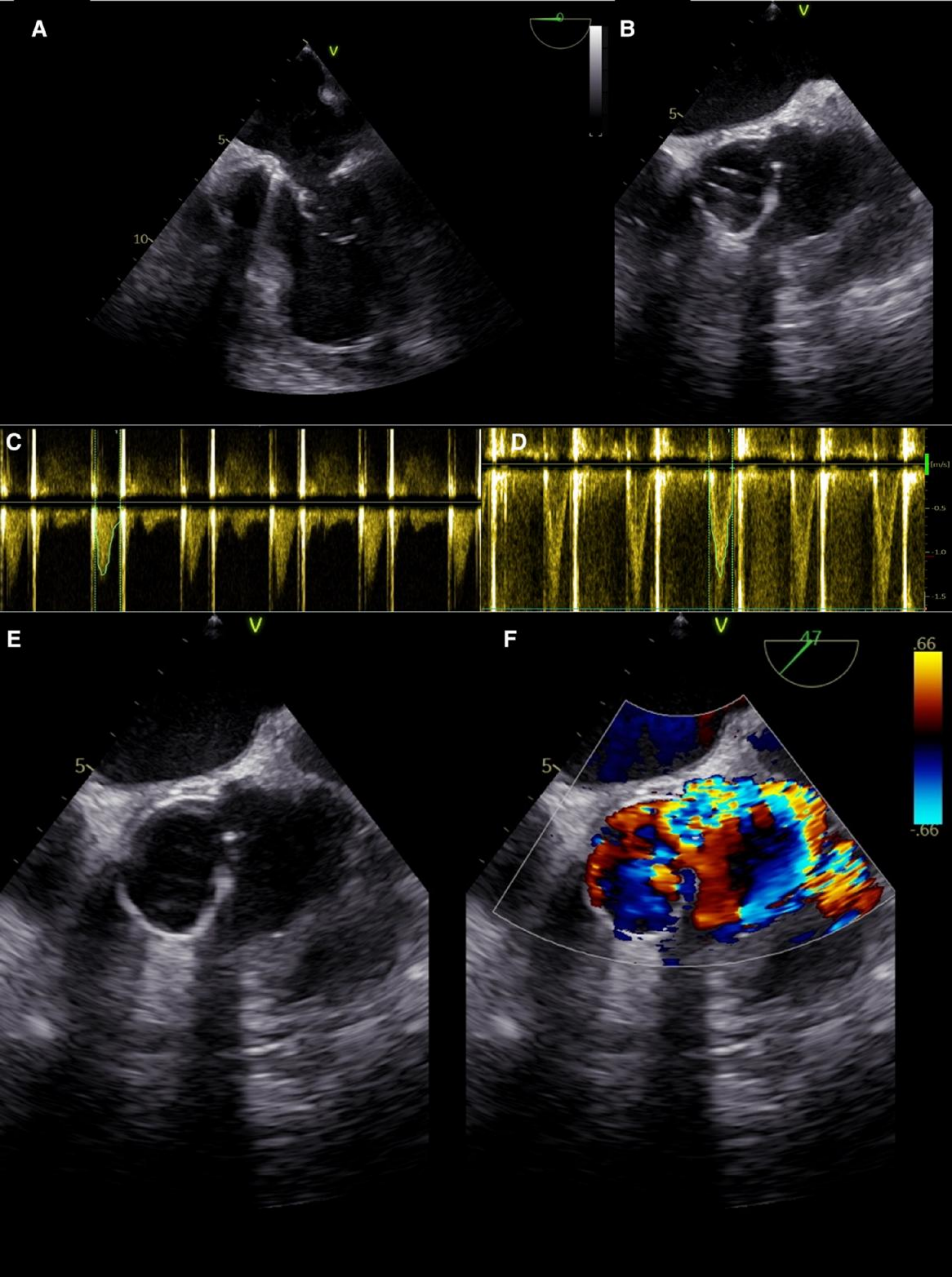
Transthoracic echocardiography and transoesophageal echocardiography images. Transoesophageal bicommisural view (*A*) demonstrating a mild to moderate dilation of the left ventricle. Transoesophageal short-axis view (*B*) showing the mechanical prosthetic aortic valve and the pseudoaneurysm of the aortic root. Continuous and pulsed wave Doppler at transthoracic echocardiography (*C–D*) demonstrating a normally functioning mechanical aortic valve (mean gradient 5.5 mmHg, max. velocity 1.7 m/s, DVI 0.7). Zoomed short-axis transoesophageal view (*E–F*) exhibiting the voluminous pseudoaneurysm with the colour Doppler demonstrating the communication of the pseudoaneurysm with the pulmonary artery with a left-to-right shunt.
